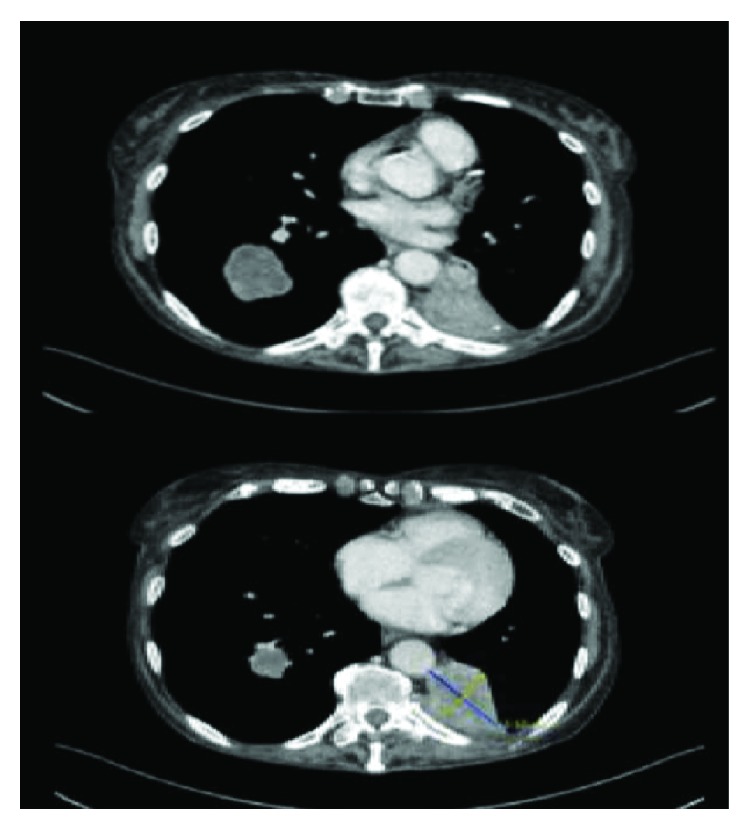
Restaging CT prior to Nivolumab. Image on the top shows the RLL at its largest diameter and the image on the bottom shows the LLL at its largest diameter.
